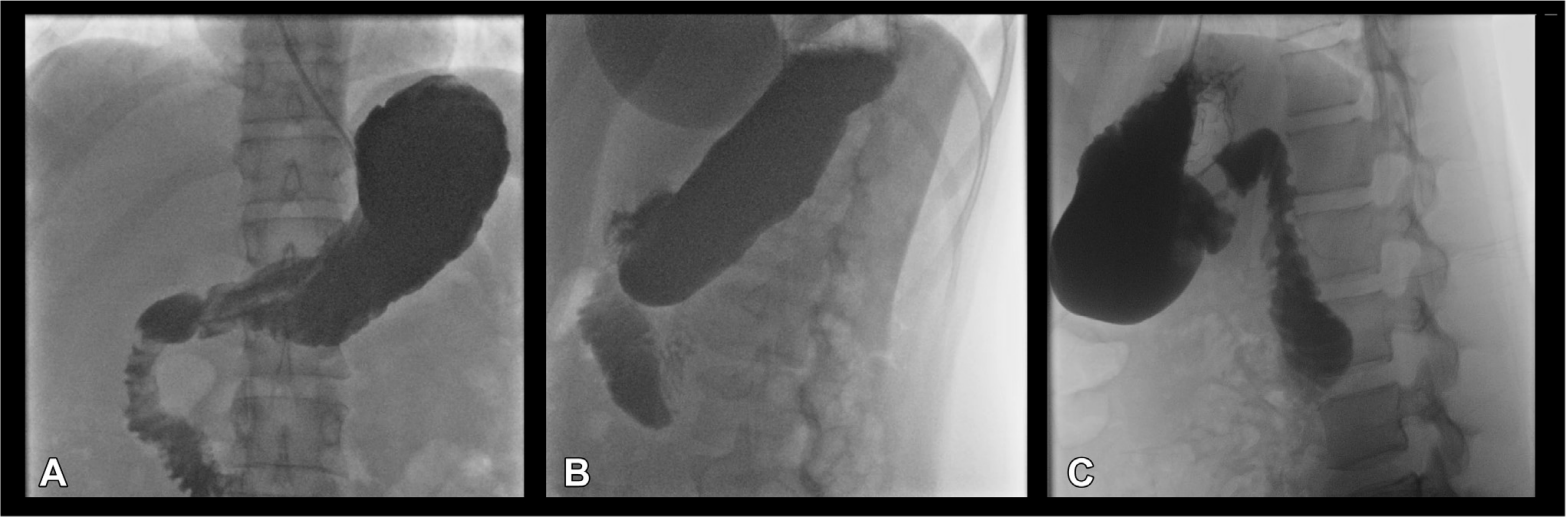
(A) Anterior (coronal view) abdominal X-ray showing the early phase of contrast with trace in the esophagus, with fall through and filling stomach fundus, body, pylorus, and duodenum, without an extraintestinal leak. (B) Lateral (sagittal view) abdomen X-ray showing contrast with later stage fall through and filling the lower part of stomach fundus, whole stomach body, pylorus, and duodenum, without an extraintestinal leak. (C) Lateral (sagittal view) abdomen X-ray showing a later stage as contrast falls through the lower part of the stomach body, pylorus, and duodenum, without an extraintestinal leak.
